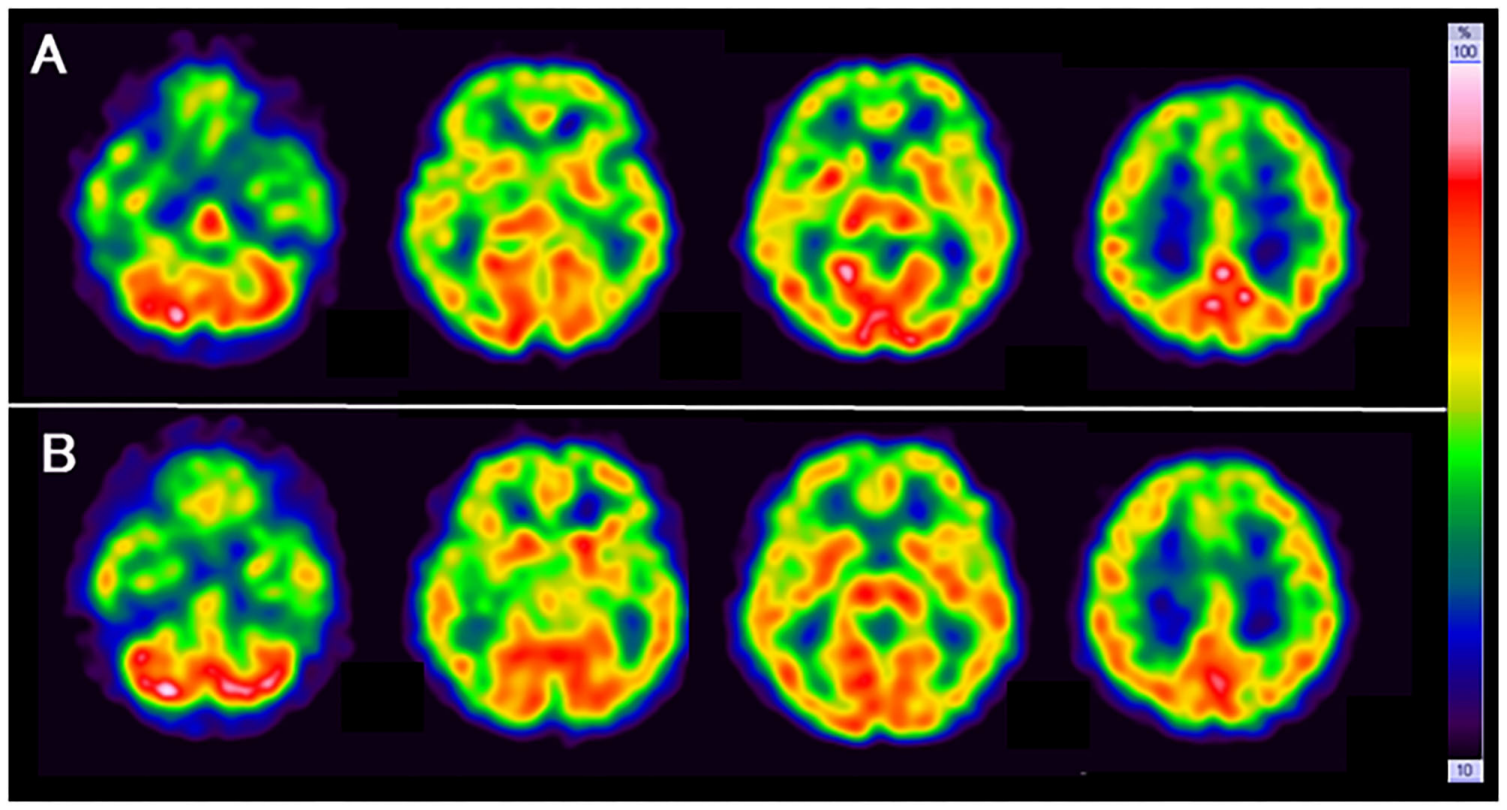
SPECT images before ECT (A, upper) and after ECT (B, lower).
